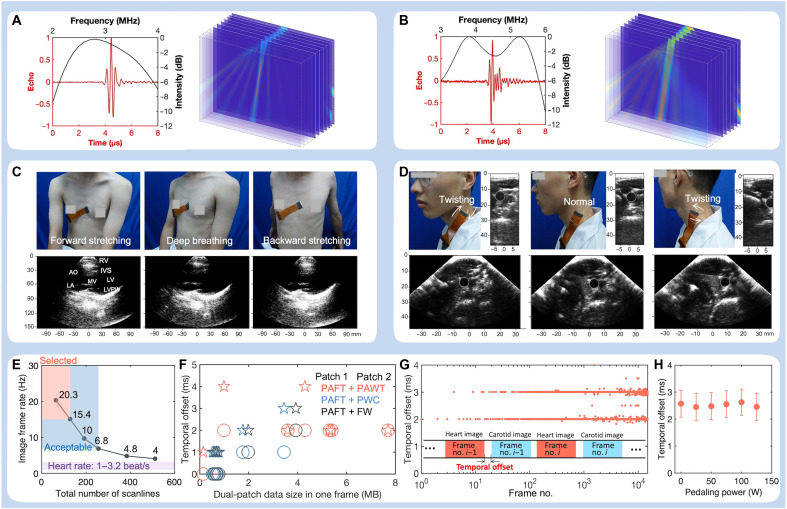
Design and characterization of the wearable ultrasound patches. (A) Pulse-echo characteristics (left) showing the center frequency (~3 MHz) and −6-dB bandwidth (~1.8 MHz, ~60%) of the cardiac patch; phased-array beam steering with focused transmissions for cardiac imaging (right). (B) Pulse-echo characteristics of the carotid patch (left), showing the center frequency of ~4.5 MHz and bandwidth of ~63% (~2.84 MHz); phased-array beam steering with wide-beam transmissions for carotid imaging (right). (C) Cardiac ultrasound images captured in response to various body postures, demonstrating stable image acquisition. RV, right ventricle; IVS, interventricular septum; AO, aorta; LA, left atrium; LV, left ventricle; MV, mitral valve; LVPW, left ventricular anterior wall. (D) Ultrasound images of the carotid artery under different postures. The plane-wave compounding imaging method (top images) fails to visualize the artery during neck twisting, whereas phased-array imaging with wide-beam transmissions (bottom images) maintains full artery visibility. (E) Frame rate for dual-patch imaging. (F) Temporal offsets for collecting cardiac-carotid image data under various combinations of imaging methods. Detailed explanation is shown in fig. S15. PAFT, phased-array imaging with focused transmissions; PAWT, phased-array imaging with wide-beam transmissions; PWC, plane-wave compounding; FW, focused-wave imaging. (G) Temporal offset measurements over 10,000 frames. The frame rate reaches ~18.6 Hz. (H) The temporal offset remained consistent across different pedaling power levels. A single-cycle burst pulse, with a center frequency defined by the patch, was transmitted for ultrasound imaging in panels (E) to (H).
